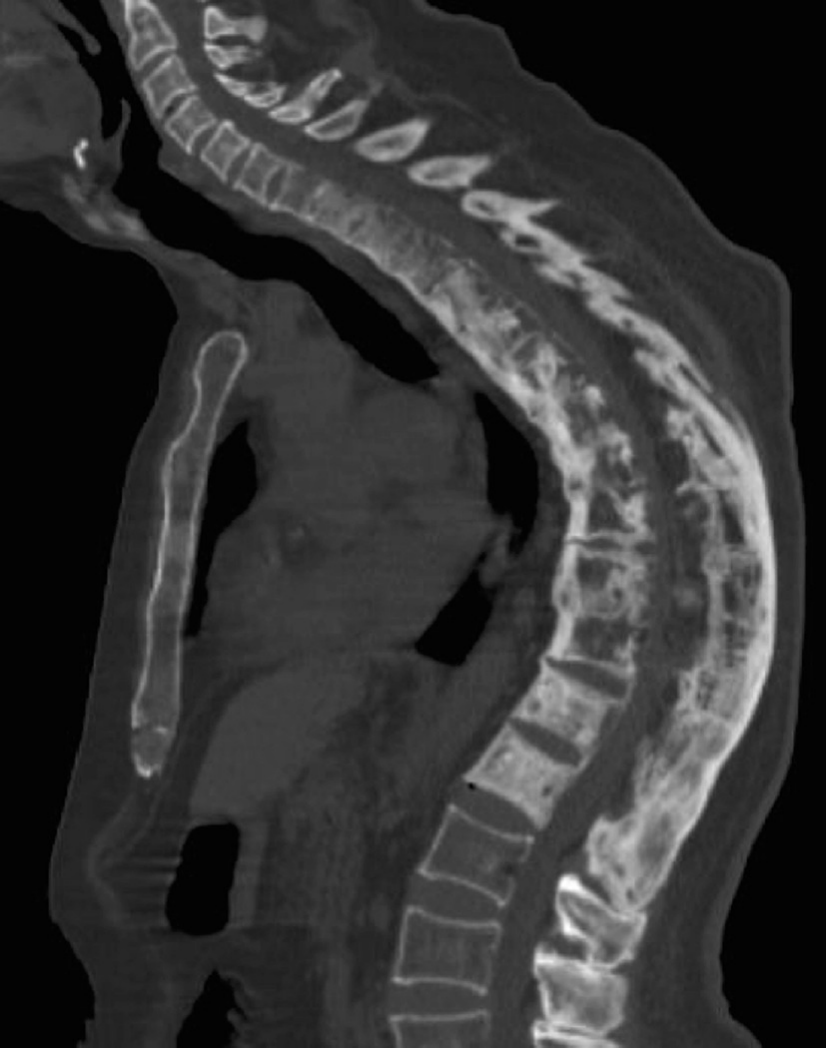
Sagittal CT reformat demonstrating typical features of ankylosing spondylitis, with extensive bridging thoracic syndesmophytes and diffuse ankylosis of the posterior elements. There is coexisting Paget’s disease, with cortical thickening, sclerosis and vertebral squaring. This produces significant kyphotic deformity and narrowing of the central canal.
